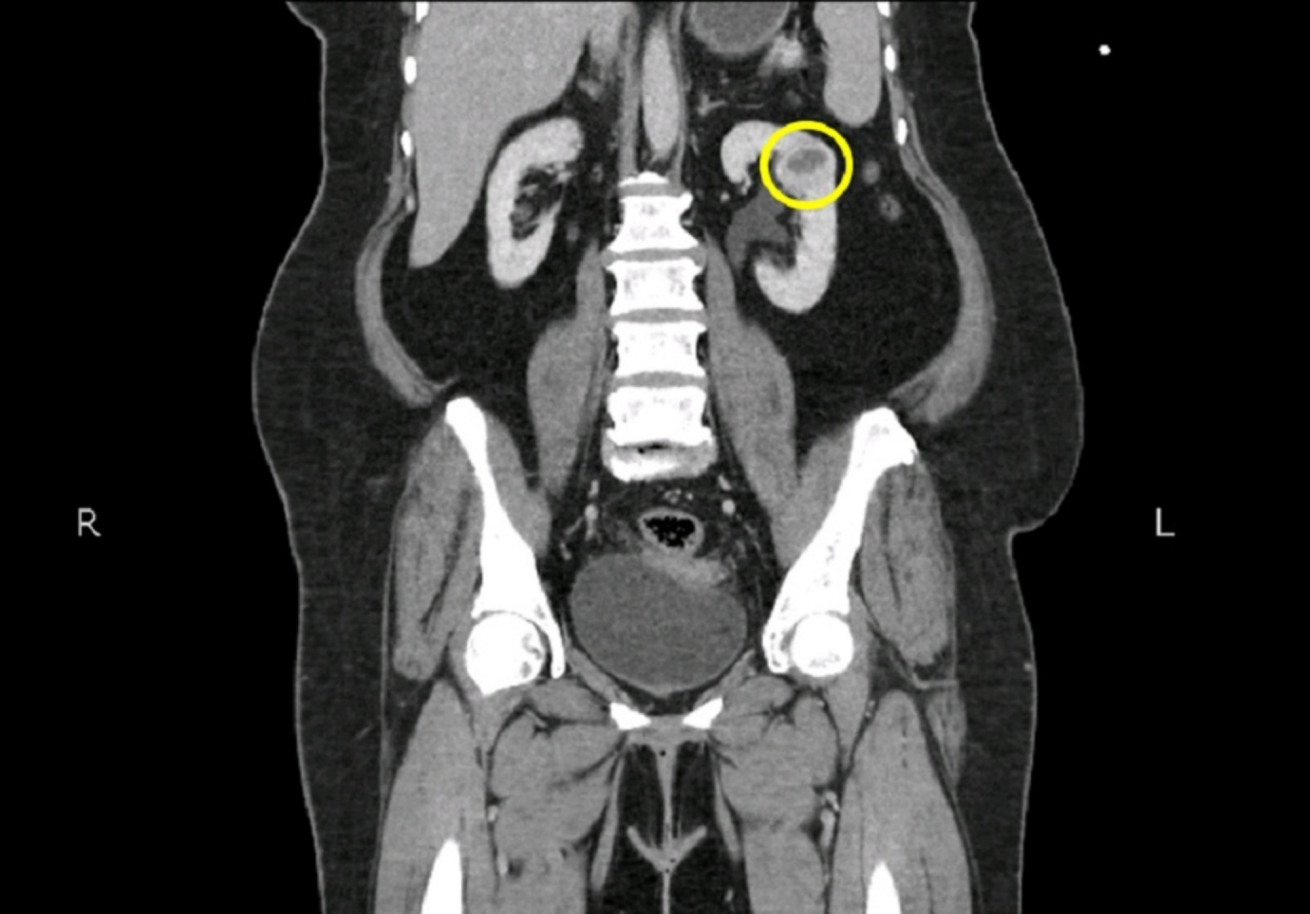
Computed tomography (CT) scan of the abdomen and pelvis (coronal view) showing a small lesion in the left kidney.
